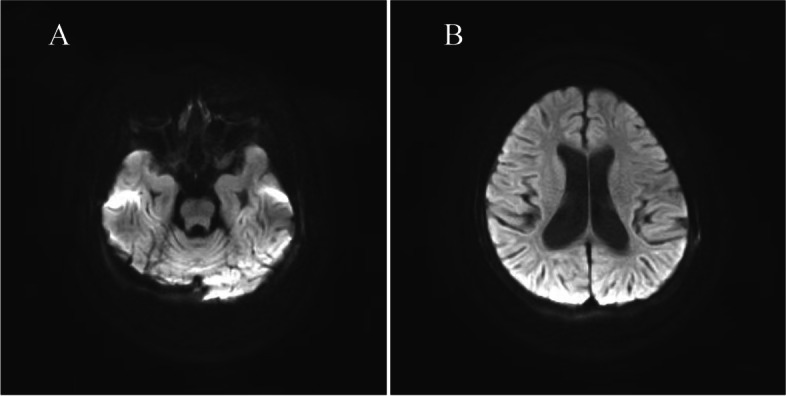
The heard MRI-DWI of the patient
